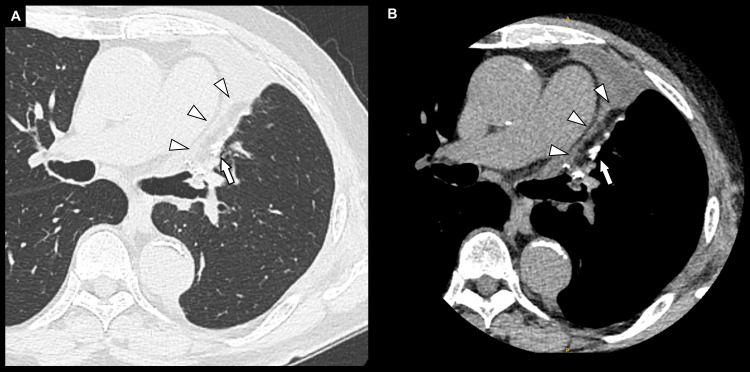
Chest computed tomography findings at eight months postoperatively. Axial views in the lung window (A) and mediastinal window (B) demonstrate favorable expansion of the left lower lobe. The anchored free pericardial fat pad (white arrowheads) is engrafted at the injury site adjacent to the staple line (white arrows).
